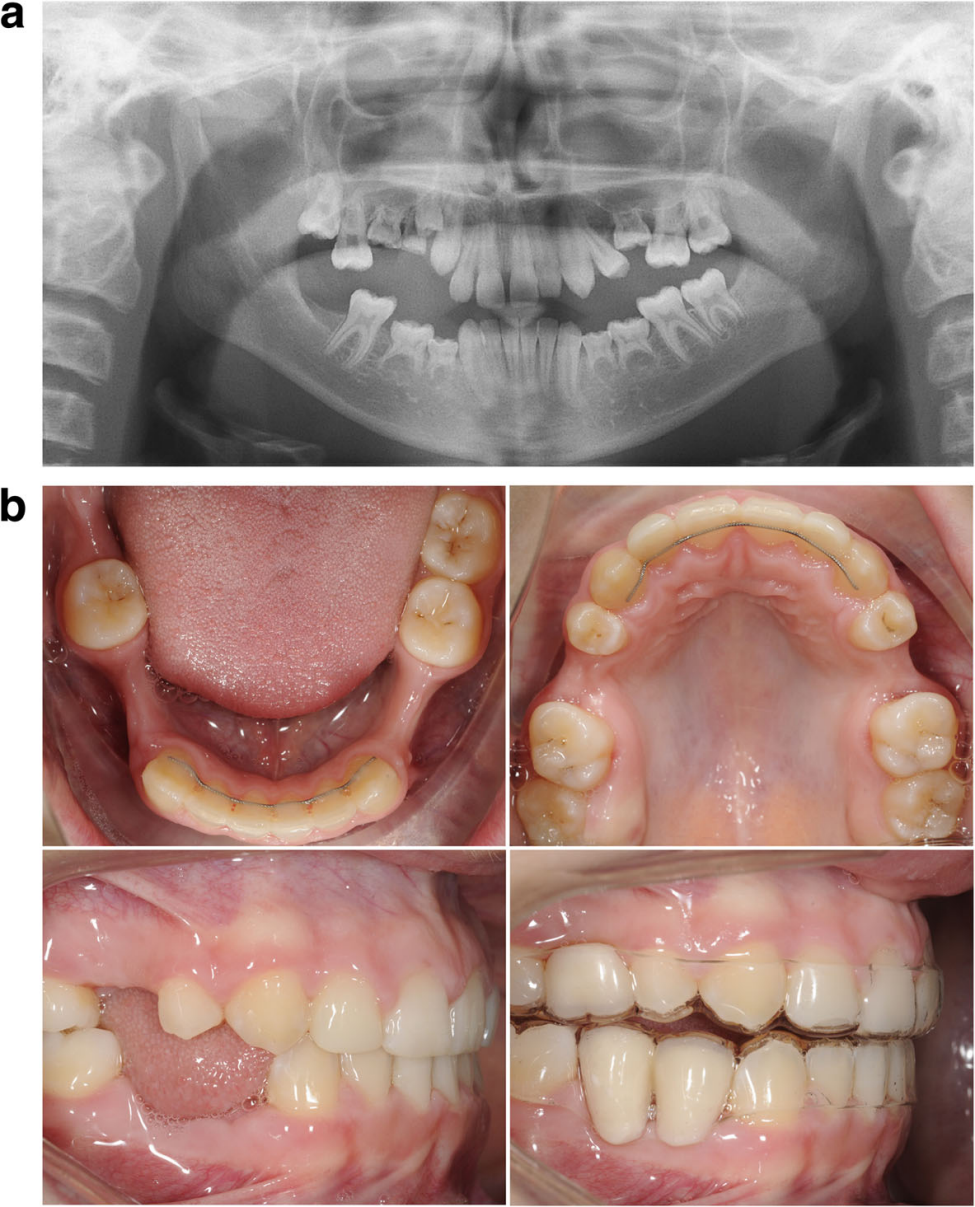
a Patient 1—orthopantomogram (OPT) at age of 13. Situation before extraction of the ankylosed deciduous teeth 55, 54, 65, 74, 75, 84, and 85 and start of orthodontic treatment. Eleven permanent teeth (including 4 third molars) were congenitally missing. b Patient 1—post-orthodontic situation at age of 16. The top of the mandibular processus alveolaris is small (*upper*). The interdental space at location of the second premolars in the maxilla is 7 and 14 mm at location of the premolars in the mandible. Six dental implants were planned (locations 15, 25, 34, 35, 44 and 45). Implant placement (inclusive bone augmentation with the autogenous retromolar mandibular bone 3 months before implant placement at the place of the 25) was postponed until the age of 18. Essix retainers were used to safeguard the width of the diastemas
